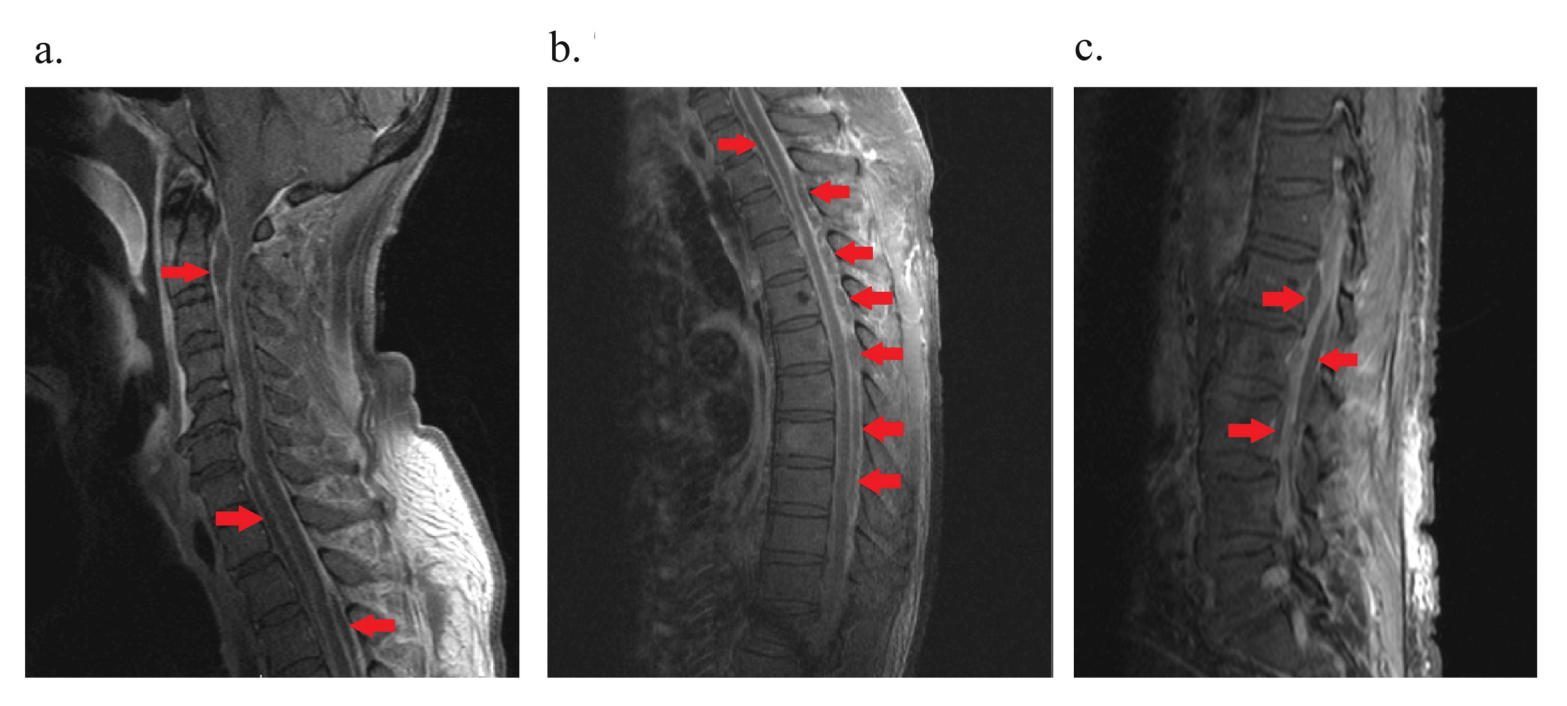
MRI of the cervical, thoracic, and lumbar spine saggital view 1a: Cervical spine; 1b: Thoracic spine; 1c. Lumbar spine The red arrows noted show various collections throughout the patient's spinal cord.
